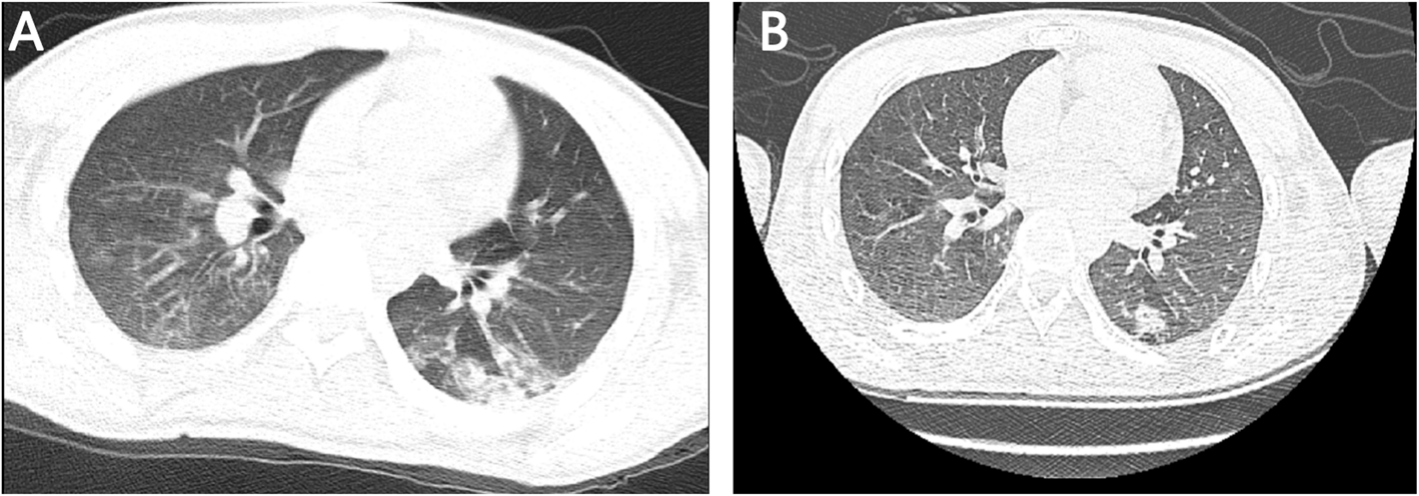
Chest CT findings. After admission, the patient’s chest CT showed bilateral pulmonary infection, especially in the inferior lobe (A). Eight days after admission, chest CT reexamination showed that bilateral pulmonary infection was controlled (B)
